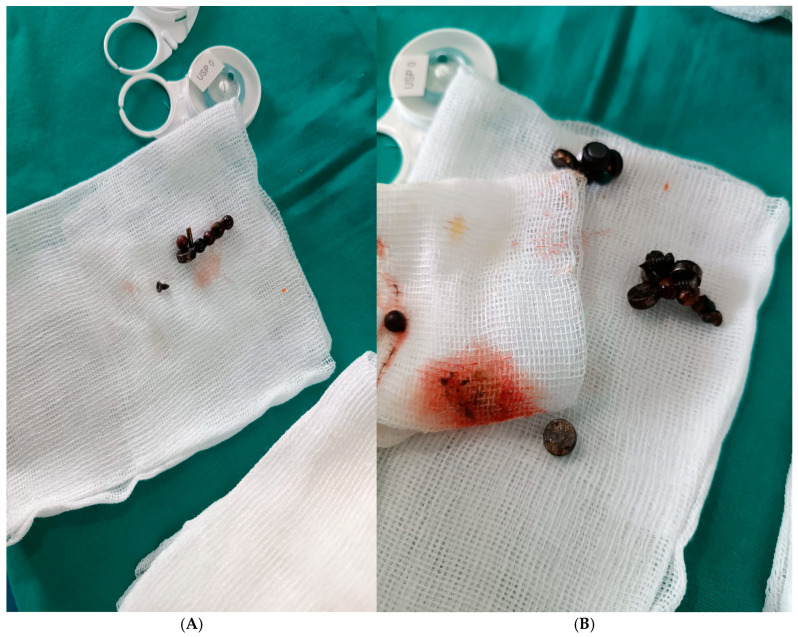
(A,B): Intraoperative retrieval of multiple ingested high-powered neodymium magnets. The magnets, some spherical and others disk-shaped, were removed en bloc from different small bowel segments following segmental enterectomy for an entero-enteric fistula. Corrosion and surface discoloration are visible, indicating prolonged gastrointestinal exposure. These foreign bodies were identified preoperatively on abdominal radiography as clustered radiopaque densities.
